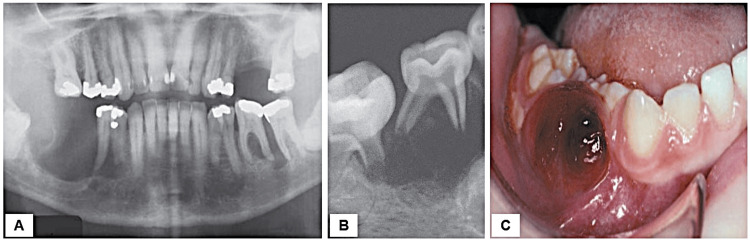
Langerhans cell histiocytosis: a) severe bone resorption involving the mandibular molar regions exhibiting similarities to advanced periodontitis; b) periapical radiograph shows marked bone resorption in the mandibular teeth in a young girl, resulting in a “floating-in-the-air” appearance; c) the lesion has extended beyond the bone producing this proliferative soft tissue mass. Permission has been obtained from the original publishers to reproduce this figure from the source [20].
